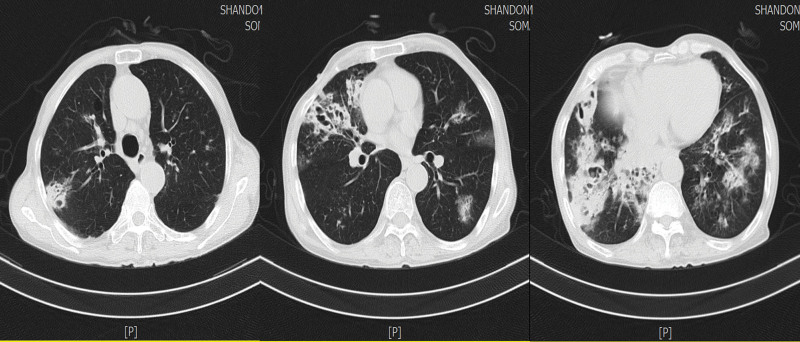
Chest CT performed on September 20, 2022 showing diffuse infiltration and bronchiectasis in both lungs, suggesting infectious lesion. CT = computed tomography.
